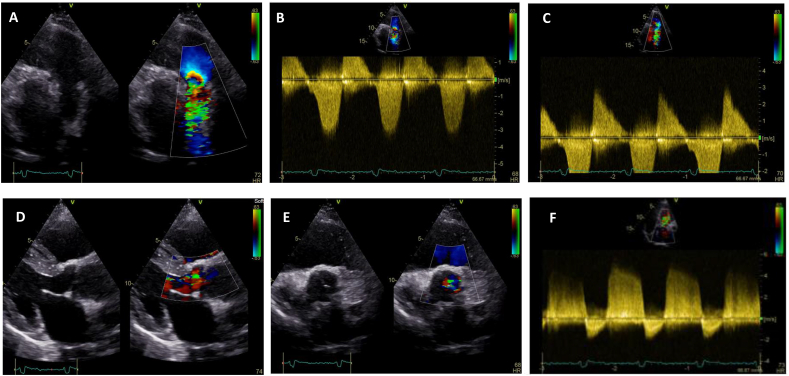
Transthoracic echocardiography showing moderate pulmonary stenosis (Vmax: 3.5 m/s, MG: 28 mmHg, PG:45 mmHg) with moderate to severe regurgitation (PHT∼170 ms) (A,B,C) and moderate aortic valve regurgitation due to mild aortic root dilation (40mm) with a central jet (PHT∼460 ms) (D,E,F).
